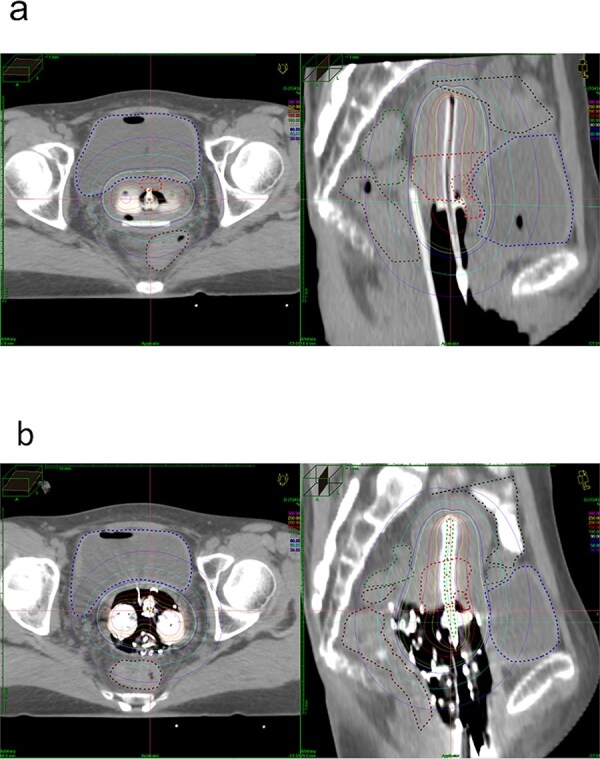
Axial and sagittal of computed tomography (CT) images of the same patient. (a) CT images taken during treatment with RR (RD2cc: 3.3 Gy, BD2cc: 5.8 Gy). (b) CT images taken during treatment with GP (RD2cc: 3.8 Gy, BD2cc: 3.3 Gy). RD2cc, D2cc of rectum; BD2cc, D2cc of the bladder; RR, rectal retractor; GP, gauze packing.
